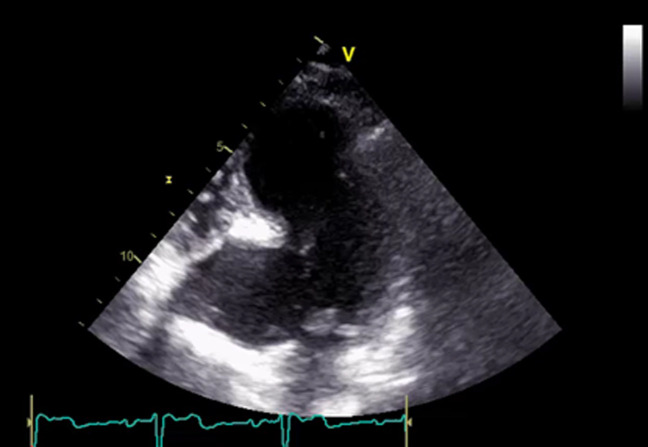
echocardiography in the apical 4 cavities view showing a giant calcified aneurysm of the basal inferoseptal wall
